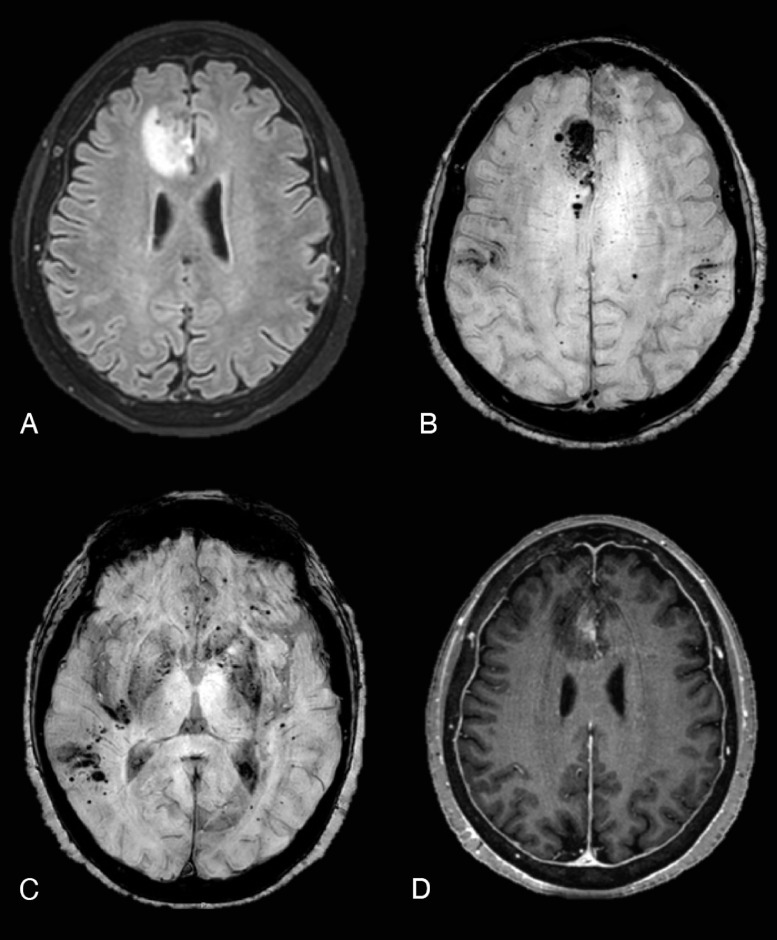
(A) FLAIR ax; (B) (C) SWI ax; (D) postcontrast T1 MPRAGE. One month later, the last MRI study showed a dramatic dissemination of new cerebral microbleeds appeared on the SWI in both hemispheres, basal ganglia, brainstem and cerebellum (B-C). FLAIR sequences (A) revealed the onset of a new area of vasogenic edema in the right superior frontal gyrus close to the pre-existing cluster of microbleeds detected in lasts controls. Dishomogeneous enhancement was seen in the same area on post contrast T1 MPRAGE (D).
